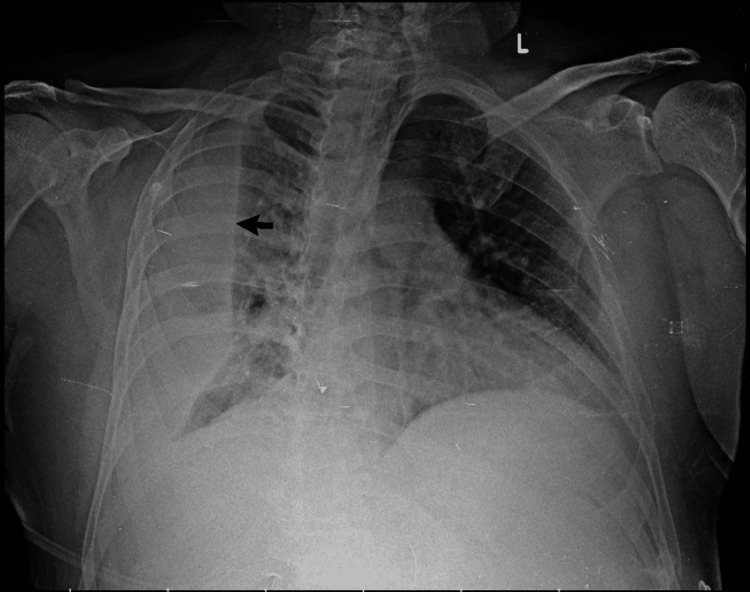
Chest radiograph post-therapeutic thoracentesis. Anteroposterior chest radiograph obtained after ultrasound-guided therapeutic thoracentesis. Despite the aspiration of fluid, there is a rapid reaccumulation and loculation of the effusion in the right mid-to-lower zones (arrow), highlighting the rapid refilling nature of the urinothorax.
